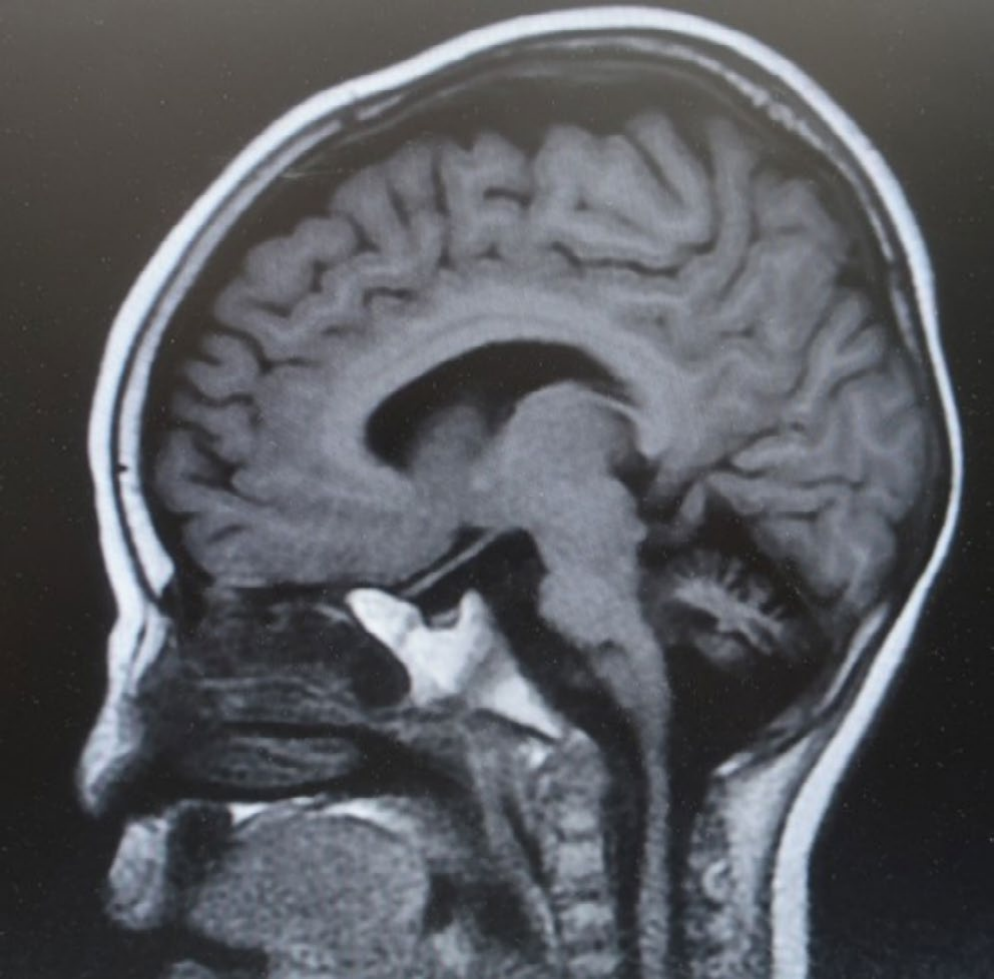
MRI image of PMM2‐CDG. This is a sagittal T1‐weighted MRI of the head of a 10‐year‐old boy with PMM2‐CDG, showing cerebellar atrophy. He suffered recurrent strokes, leading to progressive cerebellar atrophy. Additionally, developmental regression was observed in both cognitive and motor functions.
